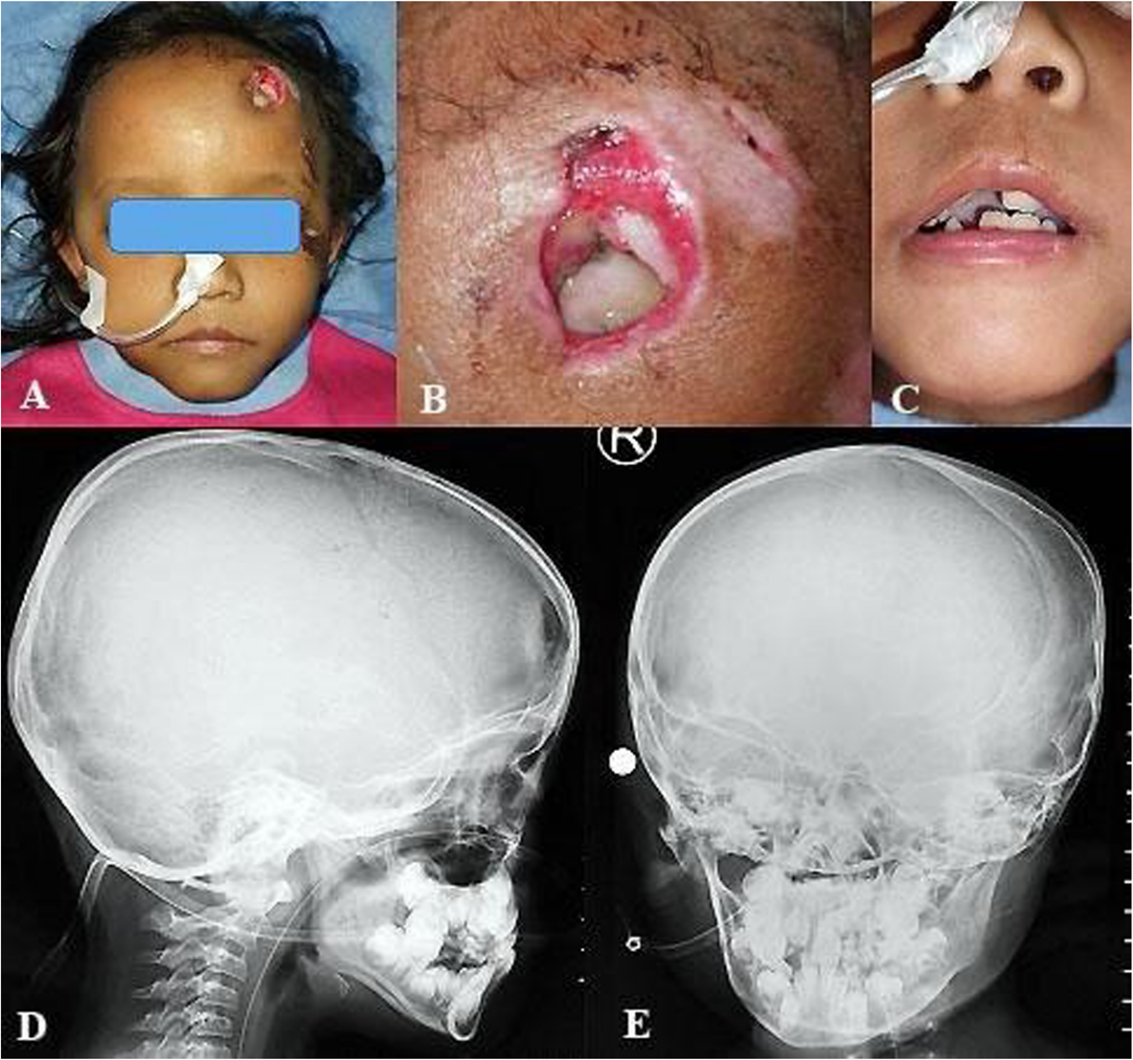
Representative images of patient condition. a There was a purulent wound at her left forehead. b Higher magnification, showing the condition of her wound. c She had a trismus with less than 1.5 cm breadth. d, e Skull X-ray demonstrating a double contour appearance at left the frontal scalp
